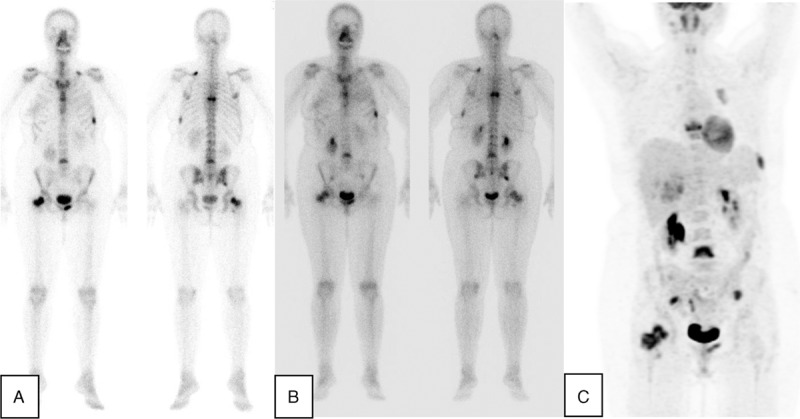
Baseline bone scintiscan with osteoblastic metastatic bone lesions in breast cancer patient (A), stable post-treatment bone scintiscan (B) and 18F-FDG-avid same metastatic bone lesions in post-treatment 18F-FDG-PET scan (C). Note: post-treatment scans were performed within 2-week interval window. 18F-FDG PET/CT = fluorine-18 fluoro-2-deoxy-D-glucose positron emission tomography/computed tomography.
